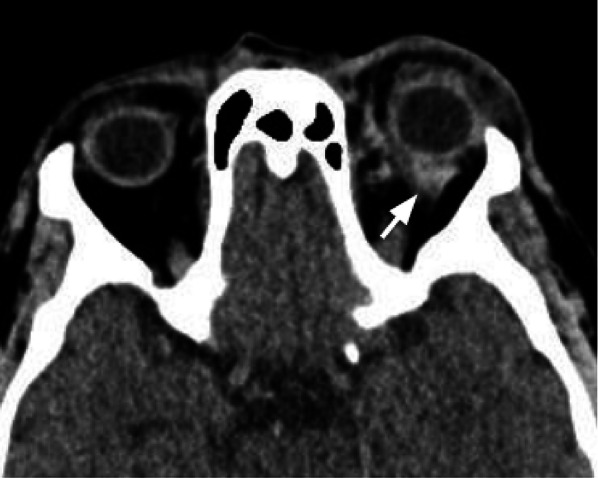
Axial CT acquisition of a retrobulbar hemorrhage visible as a retrobulbar inhomogeneous hyperdensity (white arrow). Diffuse thickening of the left palpebral subcutaneous soft tissues
